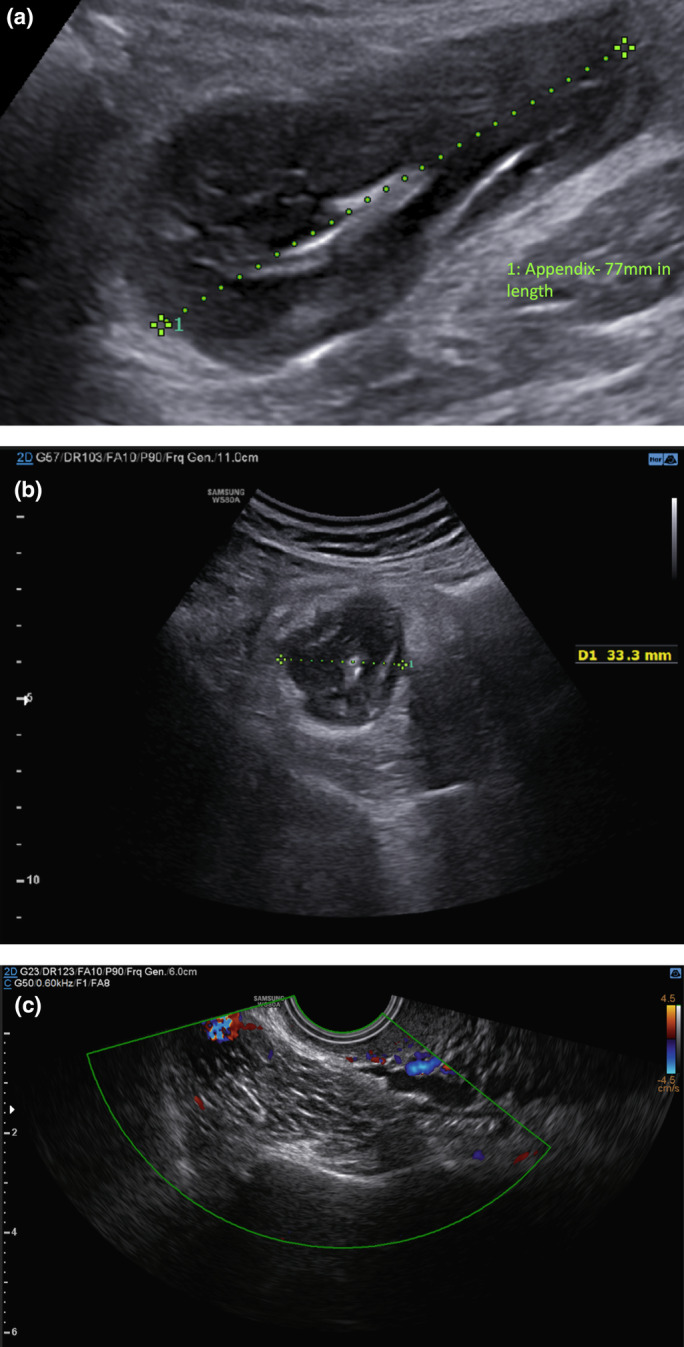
(a) Transabdominal ultrasound image of the low‐grade appendiceal mucinous neoplasm 10 weeks of post‐vaginal birth measuring 77 mm in length. (b) Transabdominal ultrasound image in transverse section of the low‐grade appendiceal mucinous neoplasm 10 weeks of post‐vaginal birth measuring 33.3 mm in width. (c) Transvaginal ultrasound image of the low‐grade appendiceal mucinous neoplasm 10 weeks of post‐vaginal birth.
